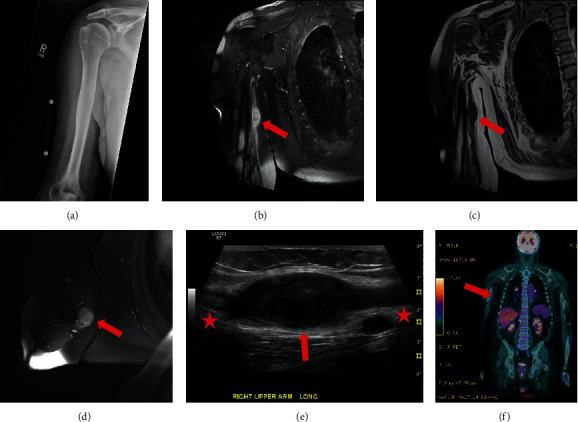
Pre- and postsurgical imaging. (a) A/P shoulder X-ray that was unremarkable. (b) Coronal short tau inversion recovery (STIR) MRI imaging showing an enhancing mass with heterogeneity (red arrow). (c) Precontrast nonfat sat T1-weighted MRI imaging showing the mass to be uniformly hypointense. (d) T1 axial fat-suppression postcontrast MRI showing heterogenous enhancing mass. (e) Image taken during US-guided biopsy showing an enlarged mass (red arrow) arising from nerve (red stars) most consistent for a PNST. (f) Postsurgical PET/CT scan showing some residual FDG signal in the postsurgical bed of the right arm (red arrow) and progression of metastatic PTC (mediastinal adenopathy, right upper paravertebral/subpleural nodule, para-aortic nodule, right medial upper lung nodule, and left upper lobe solid mass).
